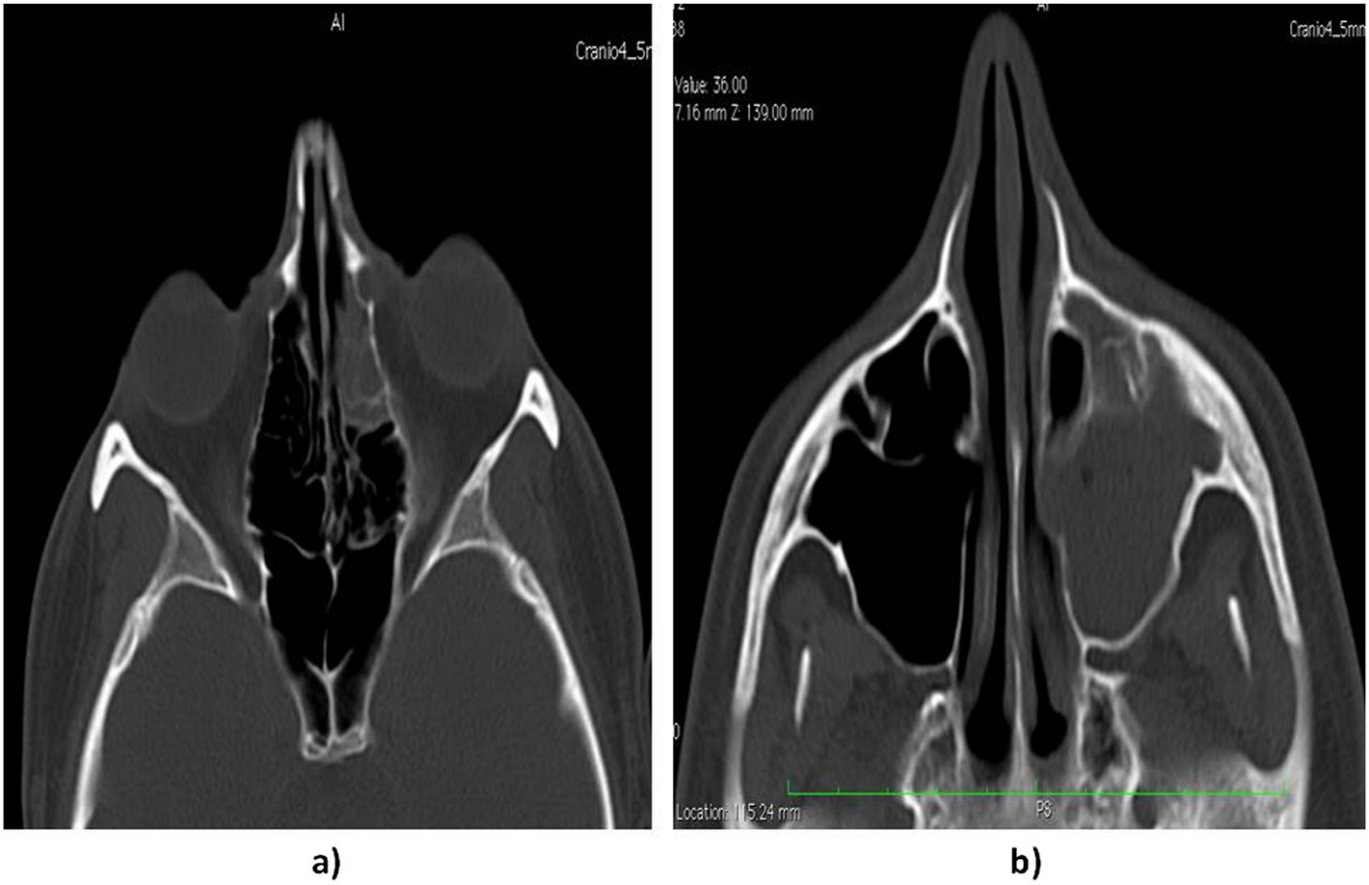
Axial computed tomography scans showing the left anterior ethmoid (a) and left maxillary sinus (b) involvement by the infective process.
